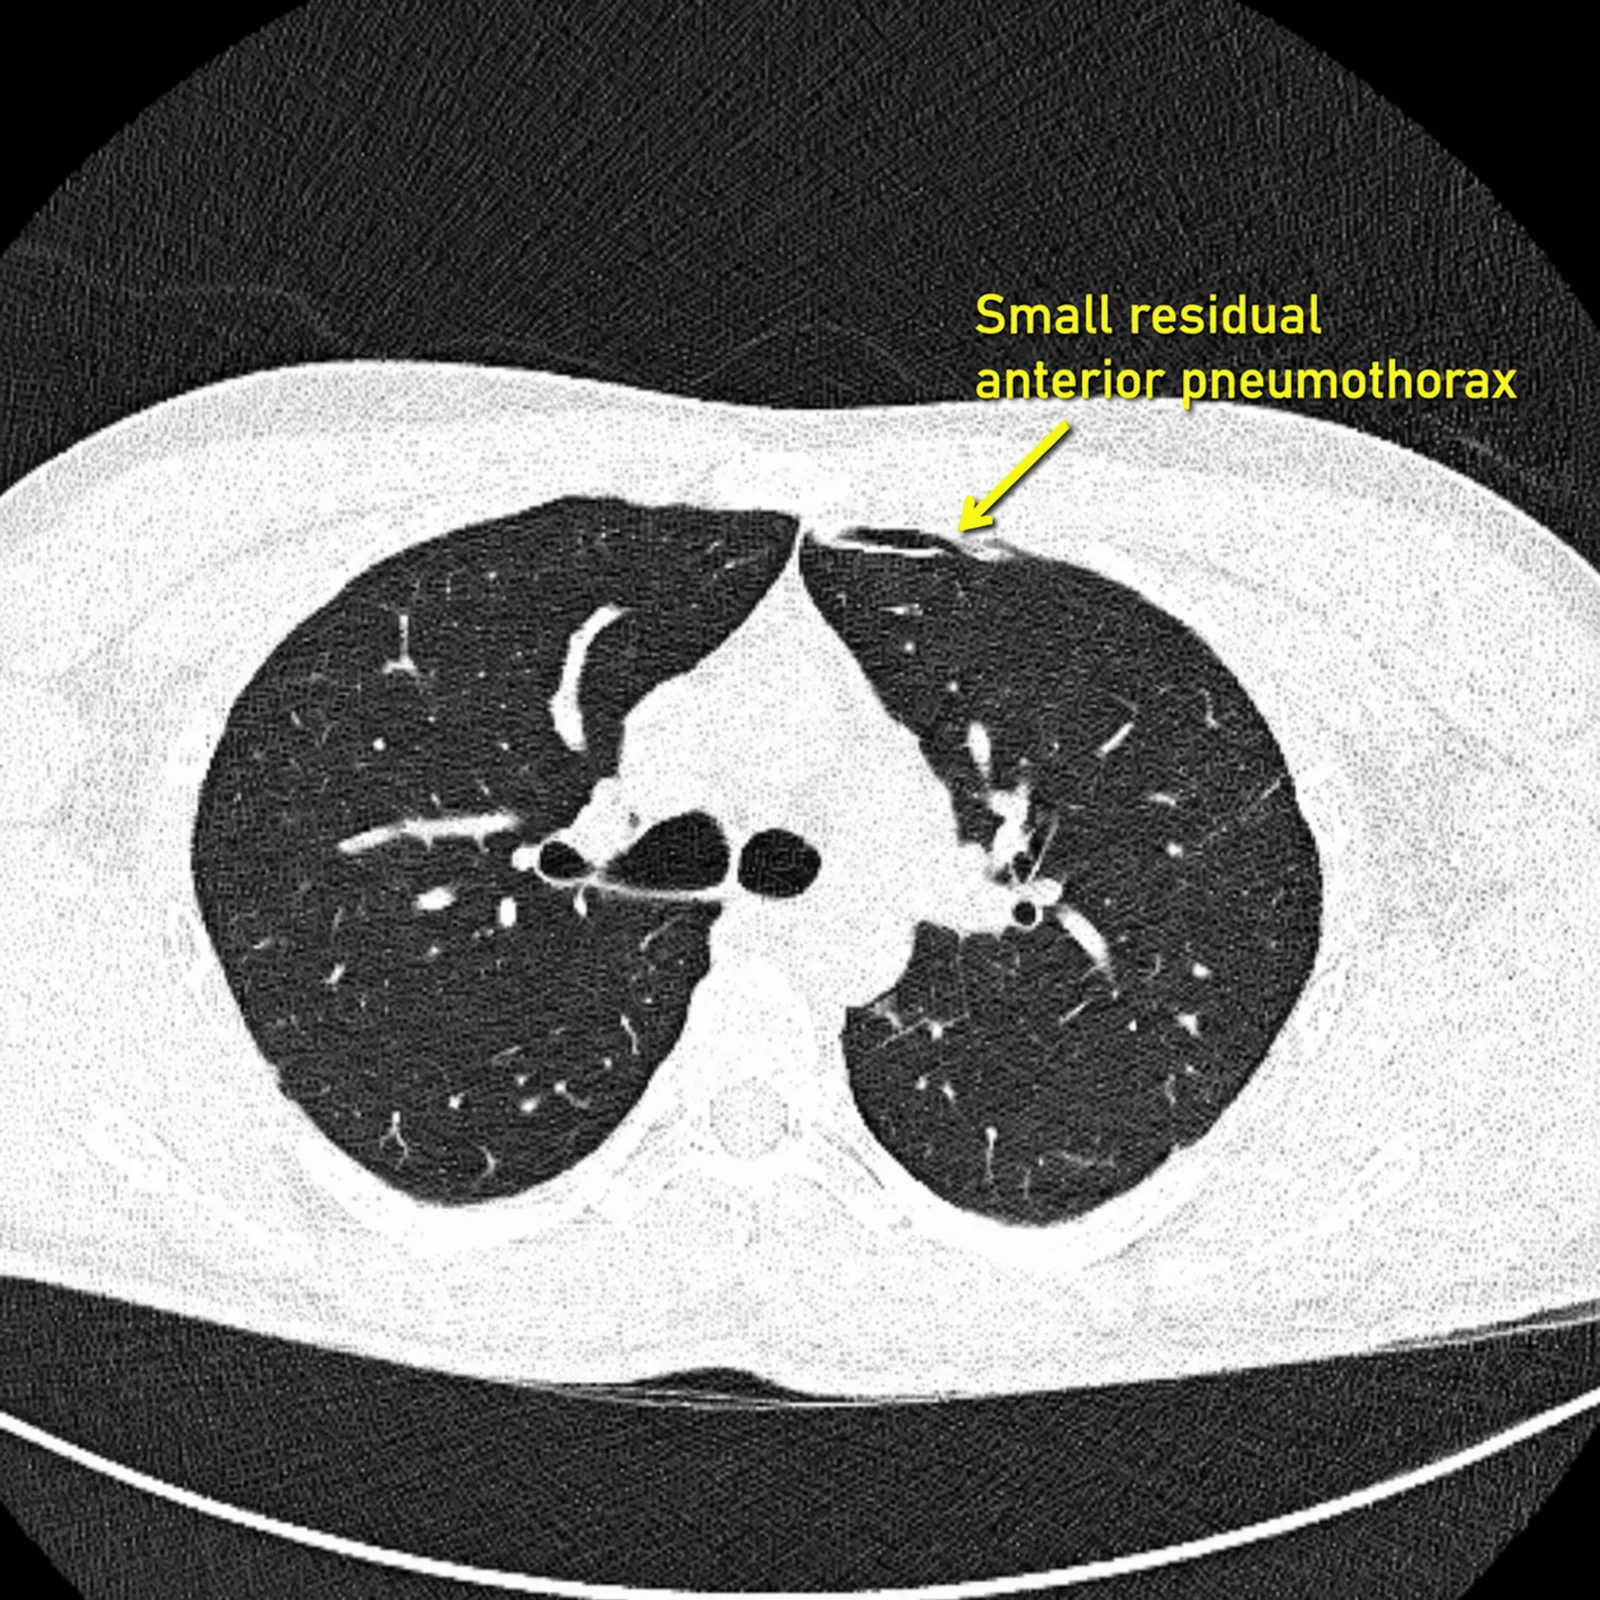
CT of the thorax: complete resolution of the left pneumothorax, a small residual right pneumothorax, and no significant structural lung abnormality The annotation identifies the residual right-sided pleural air. CT: computed tomography
